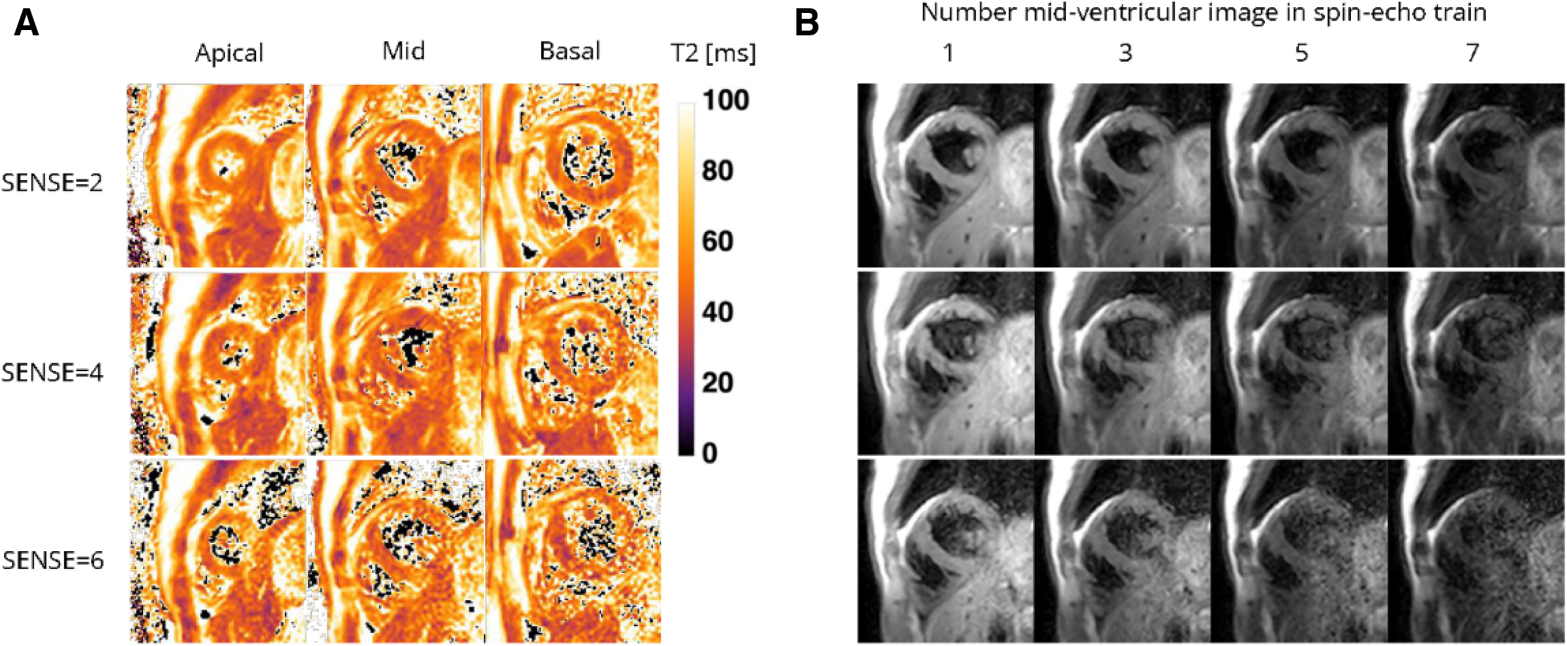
Representative (A) T2 maps and (B) T2-weighted images (echo 1, 3, 5, and 7 in spin-echo train of 8) of a healthy female volunteer acquired with the 72-channel coil using a gradient-spin-Echo (graSE) sequence with SENSE = 2, 4, and 6. Lighter colors indicate higher T2 values and darker colors indicate lower T2 values according to the color bar. ms, milliseconds.
